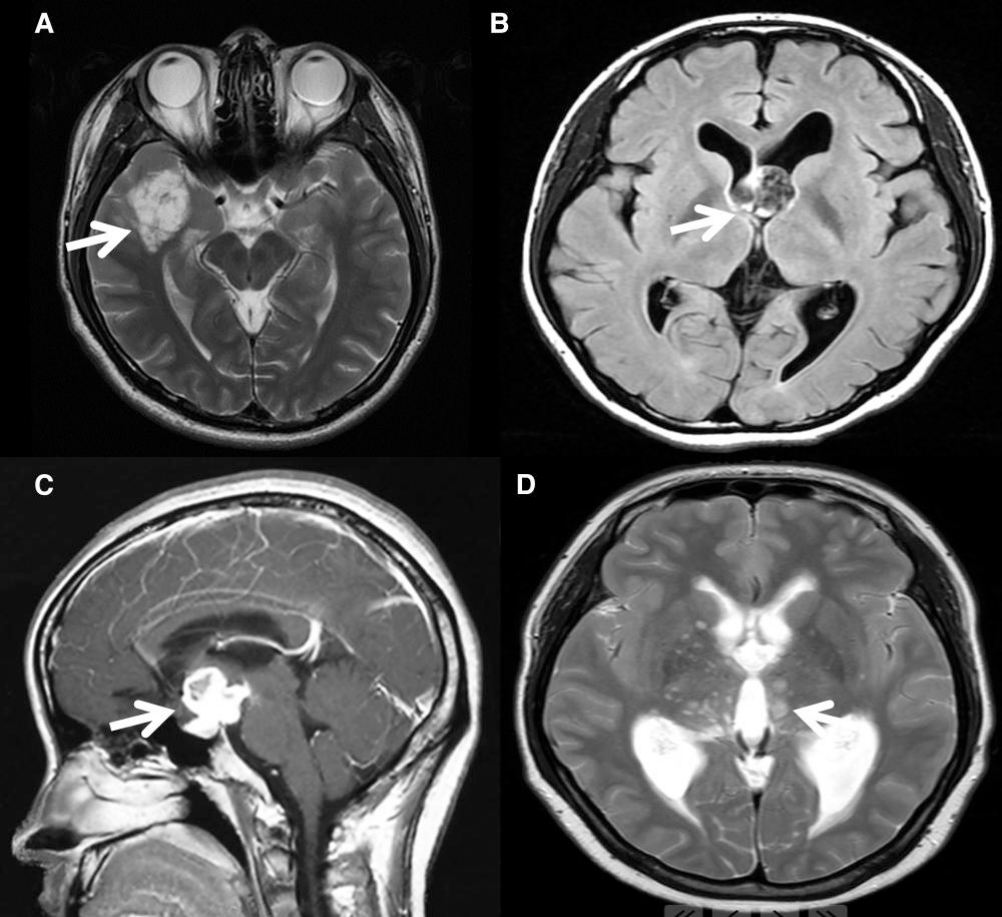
Preoperative images of representative cases in this study. (A) Preoperative MRI image of DNET-5 showing a hyperintensity on T_2_-weighted images of thickening cortex within temporal lobe without surrounding tumoural oedema. (B) Preoperative MRI image of MGNT-2 showing a well-circumscribed, FLAIR isointensity or hyperintense mass lesion in the interventricular foramen. (C) Preoperative MRI image of RGNT-3 showing a lump mass located in the sellar region with abnormal enhancement. (D) Multiple circular signals in the bilateral basal ganglia and medial temporal lobes were observed in RGNT-4. Arrows indicate the lesion.
